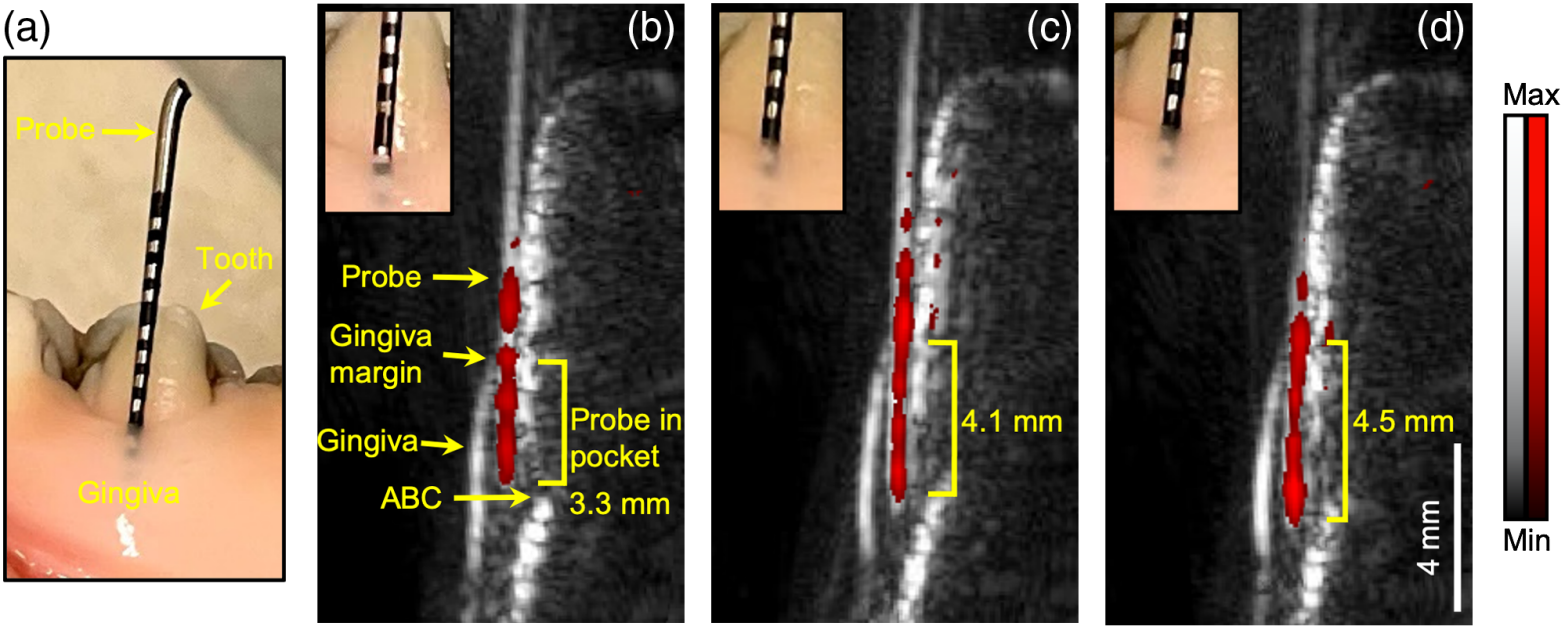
A Williams probe measures the pocket depth and offers photoacoustic contrast. (a) Williams probe in the periodontal pocket with 1-mm black tick marks on the probe. Panels (b), (c), and (d) are a stack of photoacoustic-ultrasound images when the probe is inserted into the pocket at probing depths of 3.3, 4.1, and 4.5 mm, respectively, with the hockey-stick transducer. The insets are zoomed photos of the probe in the pocket. Ultrasound image is in gray, and photoacoustic image is in red. The red line is the probe. ABC, gingiva, GM, and probe tip in the pocket are obvious.
